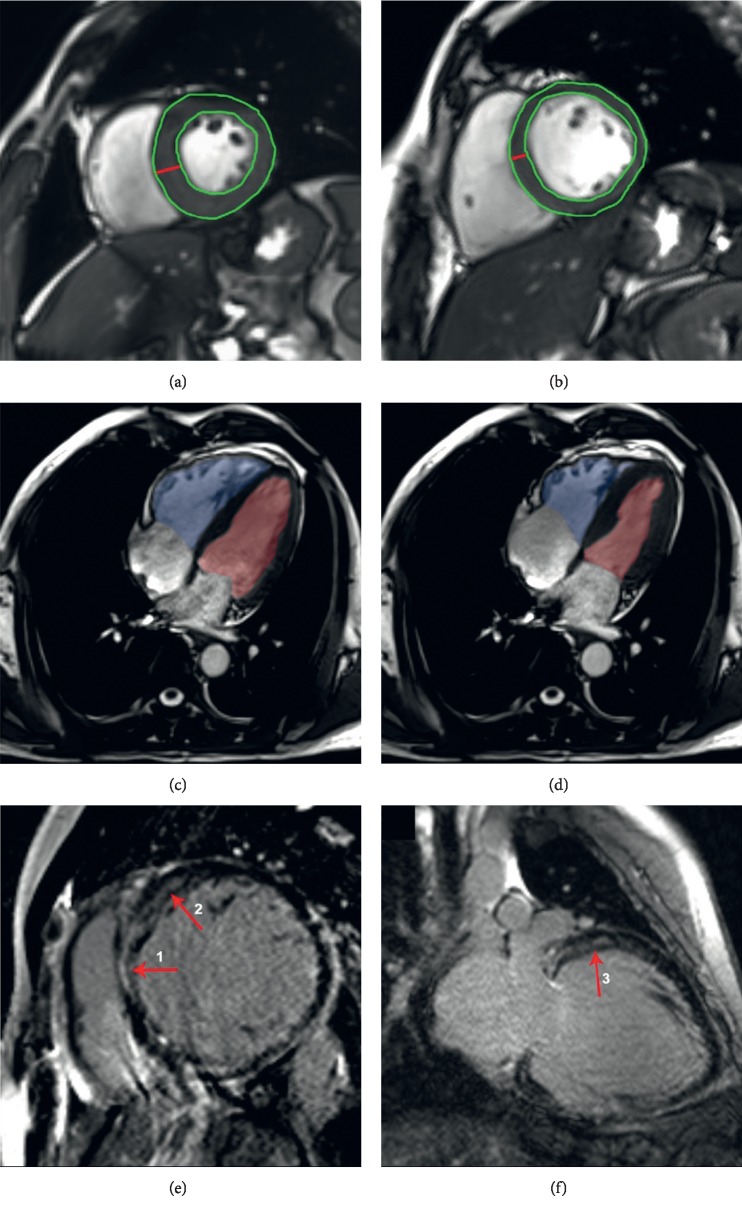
Typical cardiac abnormalities in acromegaly patients evaluated by cardiac magnetic resonance imaging. (a) Interventricular septum (IVS) hypertrophy and left ventricular (LV) hypertrophy shown. (b) Normal IVS thickness and LV mass index. The red lines shown in (a) and (b) indicate the lines along which IVS thickness was measured, and the areas surrounded by green lines reveal the LV mass used for calculations. (c) LV and right ventricular (RV) systolic dysfunction at end-diastolic stage. (d) LV and RV systolic dysfunction at end-systolic stage. The images shown in (c) and (d) were recorded from the same patient. The red area represents the LV volume, and the blue areas represent the RV volume. (e) Mid-wall late gadolinium enhancement (LGE) of the IVS myocardium (red arrow “1”) and the LV myocardium on the cardiac short axis (red arrow “2”). (f) Mid-wall LGE of the basal anterior LV myocardium on the cardiac long axis (red arrow “3”).
